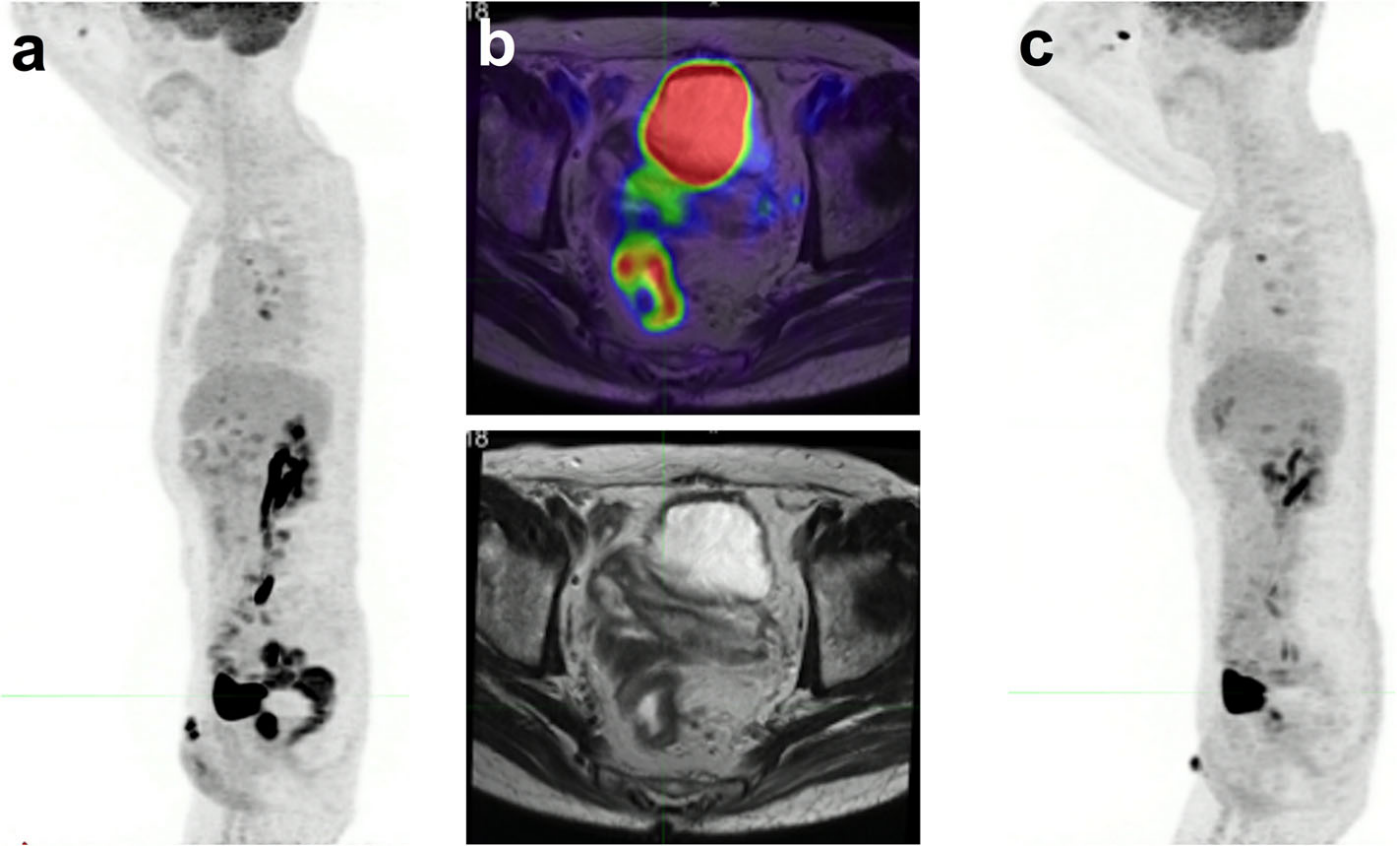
Example of metformin effect. At baseline (a) metformin obscured uptake in a known rectal cancer. The baseline uptake in the primary tumour is demonstrated on the fused PET/MRI (b) but adjacent uptake in normal bowel compromised assessment of disease extent. The post-treatment scan (c) done with metformin withdrawal for 48 h demonstrated a complete metabolic response, which would otherwise have been impossible to discern
